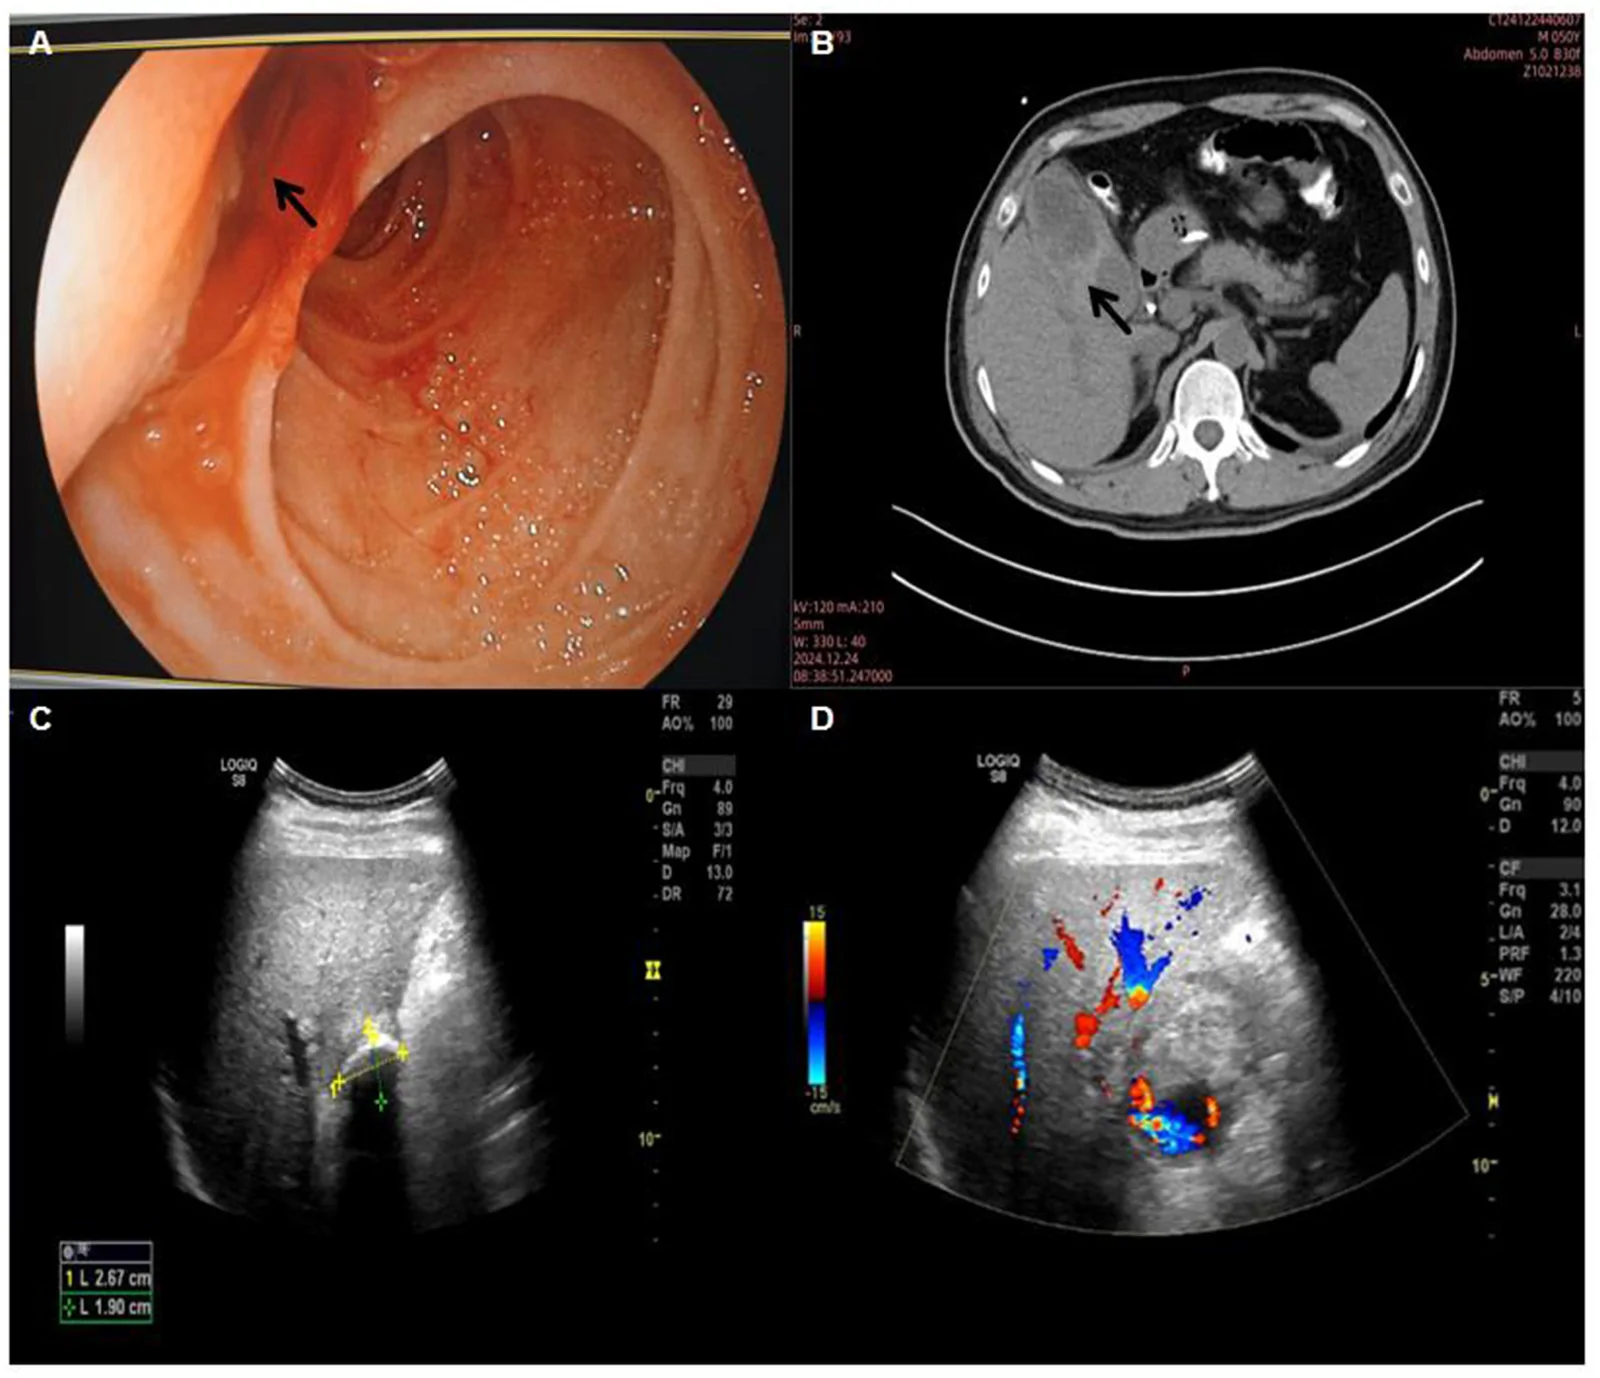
(A) Endoscopic examination revealed active oozing from the duodenal papilla (arrow), without a distinct bleeding point; however, bright red refluxing blood was evident. (B) Non-contrast computed tomography (CT) demonstrated an enlarged gallbladder with thickened walls and a slightly hyperdense area in the gallbladder (arrow), which may indicate the presence of blood components. (C) Ultrasound displayed an irregular anechoic region at the neck of the gallbladder. (D) CDFI demonstrated abundant arterial blood flow.
